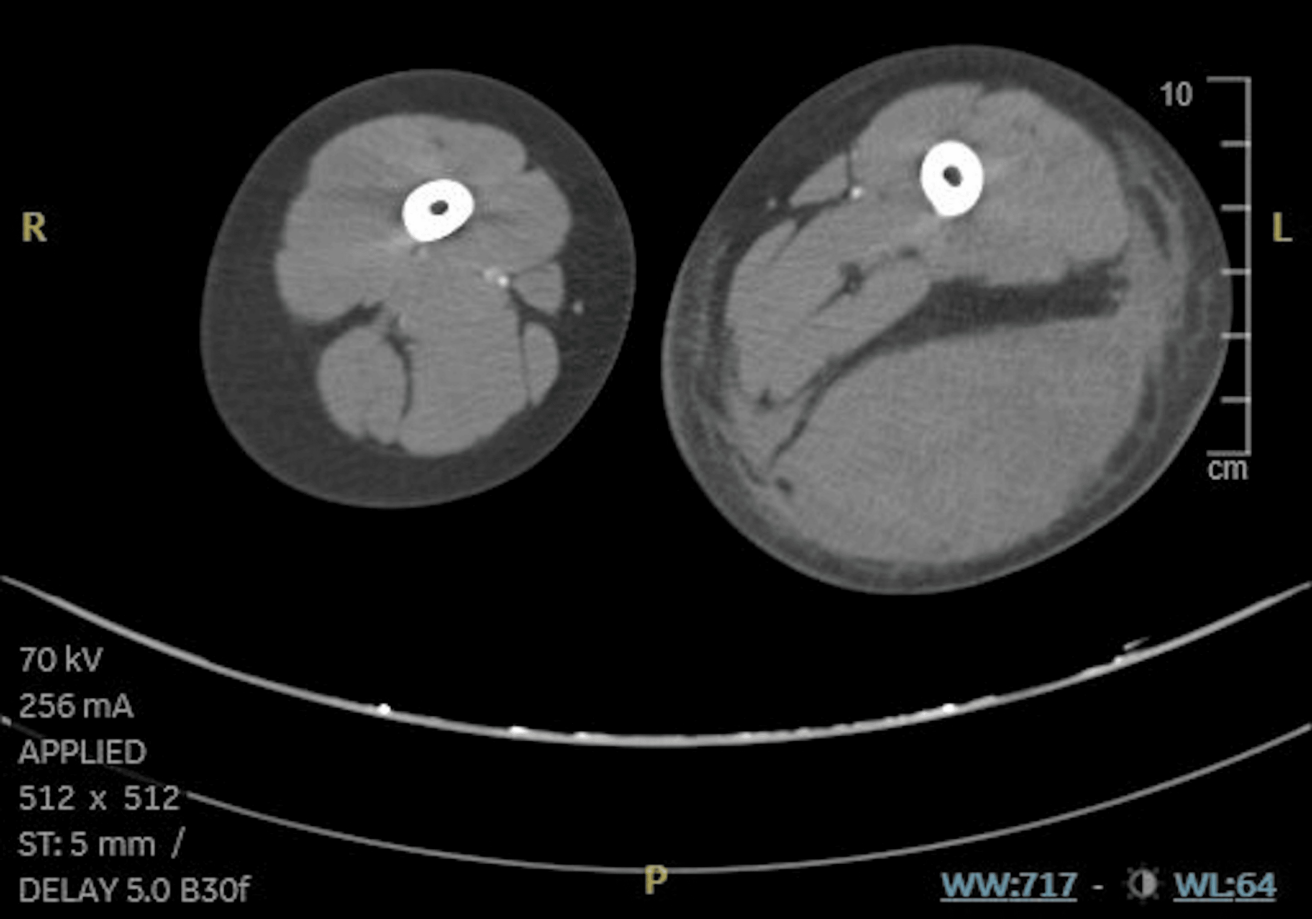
CT showing prominent subcutaneous and intermuscular hematoma in the left thigh
